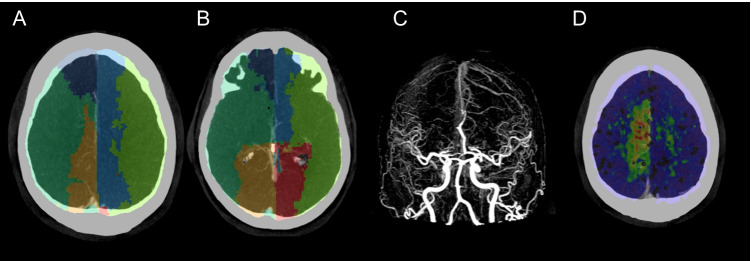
Right ACA occlusion. Occluded A2 segment of the right ACA. A, B Axial slices of CTA with VTM overlay. C MIP CTA demonstrates a hypoplastic right A1 and an absence of distal opacification in the right ACA territory. D CT perfusion (Tmax). VTM software-derived vascular regions show a reduced right ACA region (dark blue) with an equal increase of both MCA and PCA regions on the ipsilateral side. CT perfusion demonstrates a penumbral region within the right ACA territory. When correlating CT perfusion with VTM, the delayed region on Tmax is located in the VTM region of ACA that has been replaced by MCA and PCA collaterals
